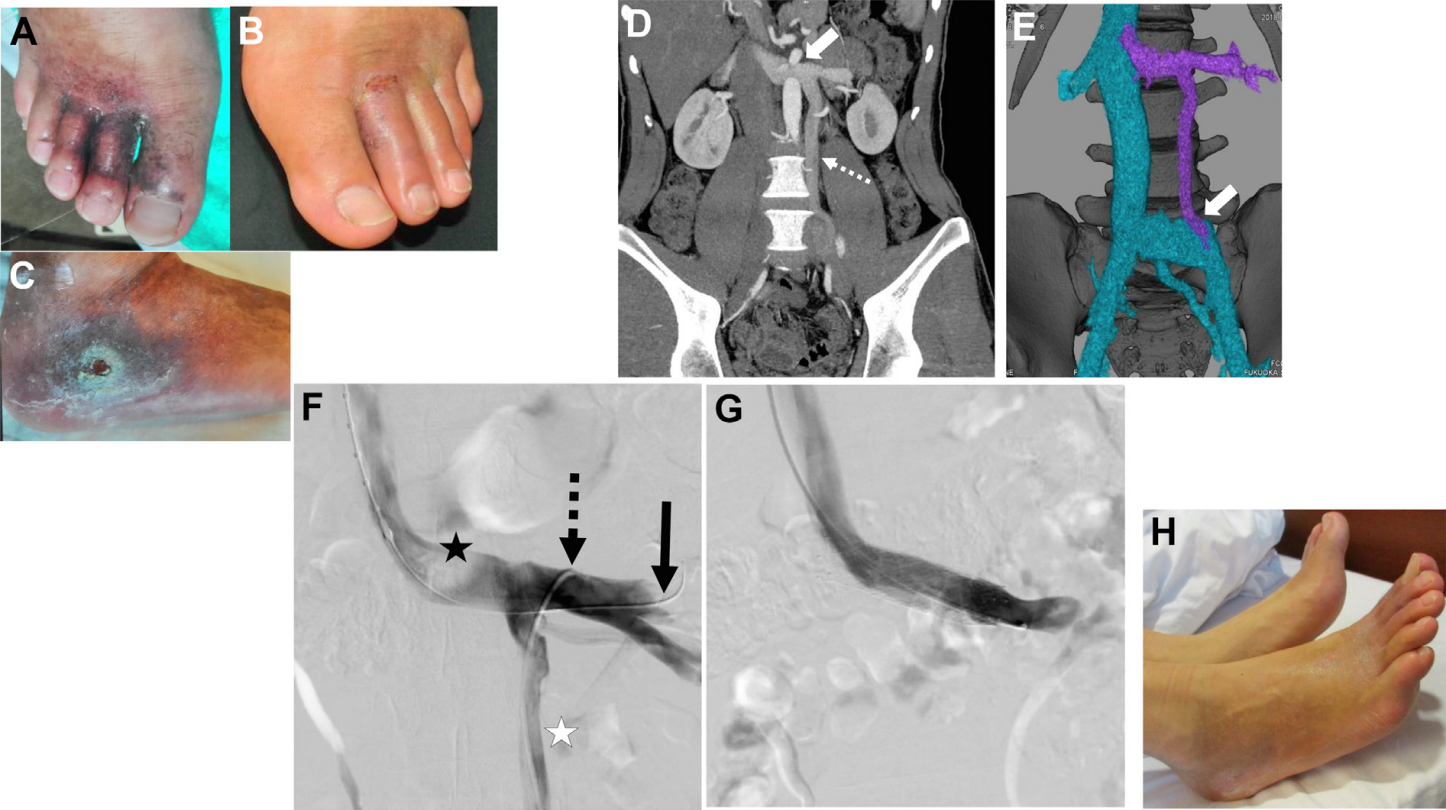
Case 3. (A-C) Preoperative photograph of the patient demonstrating ulcers over the lateral ankle and forefoot bilaterally. (D, E) Computed tomography angiography (CTA) demonstrates compression of the left renal vein (LRV) (D, white arrow), and dilatation and early opacification of the collateral vein (D, dashed white arrow), which communicate with the left common iliac vein (CIV) (E, white arrow). The collateral vein could be described as a duplication of the inferior vena cava or ascending lumbar vein rather than the left gonadal vein (LGV). (F) Venogram showed stenosis of the LRV with contrast attenuation over the abdominal aorta (black star) and large refluxing drainage pathway (white star). The LRV could be approached from the right internal jugular vein (via the inferior vena cava) (black arrow) and the left common femoral vein (FV) (via the drainage vein) (dashed black arrow). (G) After LRV stenting, the collateral pathway has disappeared and the LRV is well opacified. (H) Three-month postoperative photograph showing healing of the skin ulcers.
